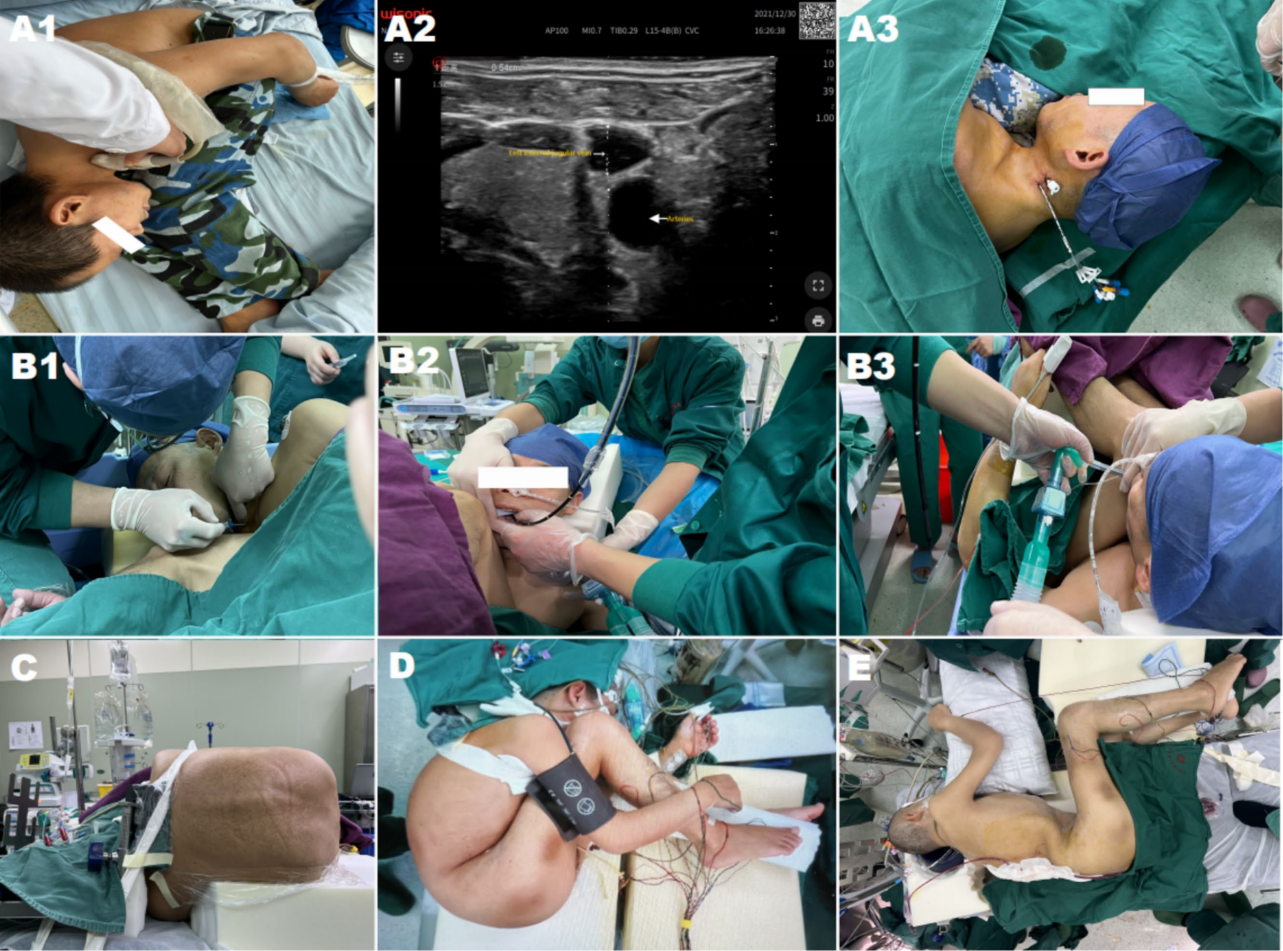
(A1–A3) Display the ultrasonic evaluation of blood vessels and establishment of a central vein. (B1–B3) Exhibit awake endotracheal intubation was performed after ultrasound-guided cricothyroid membrane puncture and tracheal mucosal anesthesia before endotracheal intubation. (C) Shows surgery was performed in a lateral position. (D) Depicts basic intraoperative vital signs and hemodynamic monitoring. (E) Expose the patient corrected the posture after the first-stage surgery.
